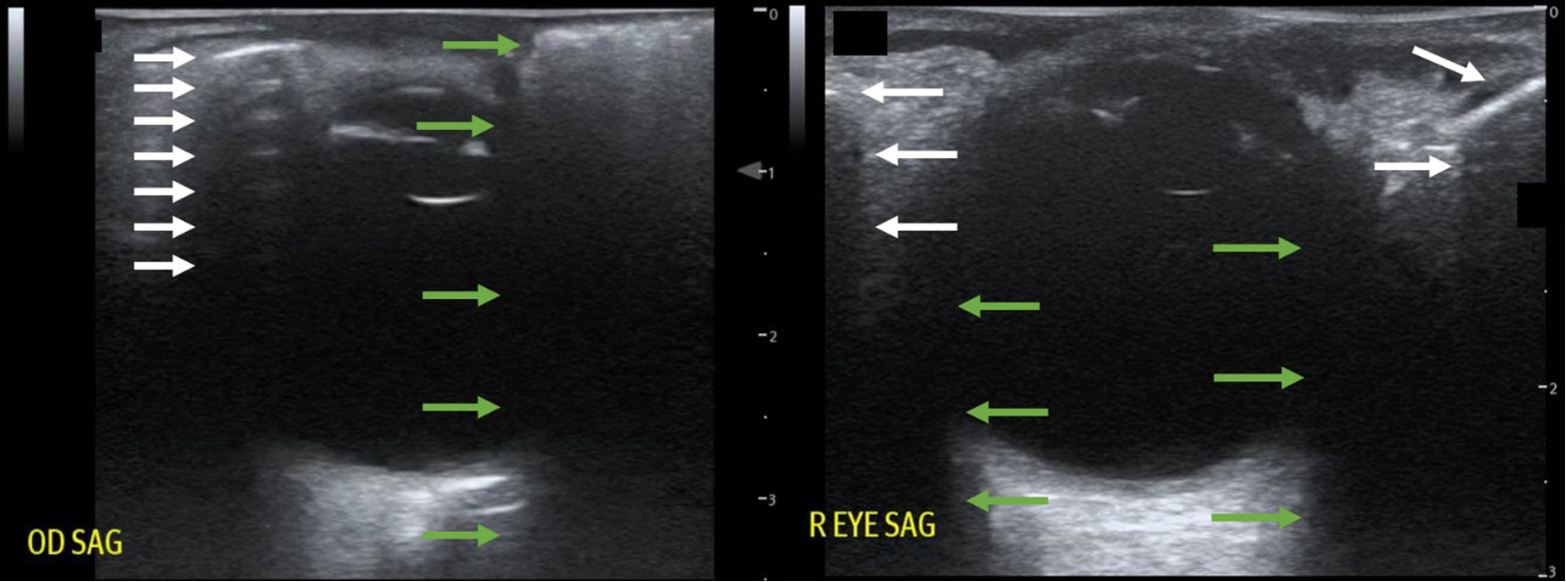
Point-of-care ultrasound of the patient’s eye (left) compared with a normal eye ultrasound (right). In the patient’s ultrasound on the left, a hyperechoic line is seen just below the skin surface (top white arrow), which represents the air-tissue interface of subcutaneous emphysema. Below are equally spaced, repeating lines of reverberation artifact (additional white lines), typical of highly reflective air-tissue interfaces, analogous to “A-lines” on normal lung ultrasound. Comparatively, in the right image, the shadow of the bony orbit in a normal eye ultrasound (white arrows) originates deeper below the skin surface, is sharply demarcated at the edges, and lacks reverberation artifact. Also shown in the patient’s ultrasound on the left is the “dirty shadowing” associated with subcutaneous air (green arrows.). Compared to the shadows of edge artifact indicated by the green arrows in the normal ultrasound on the right, these “dirty shadows” originate from an irregular surface, appear more “smeared,” and are not well-demarcated at the edges.
